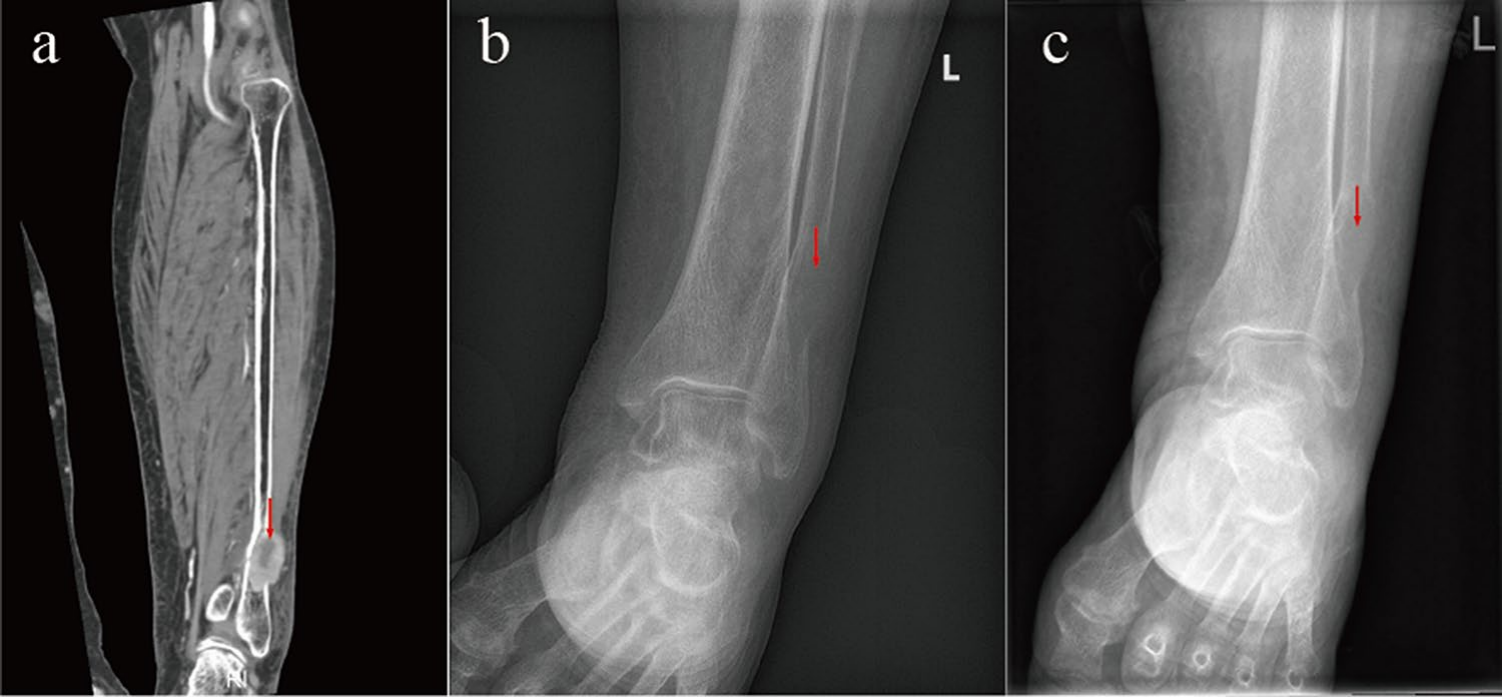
Imaging examination for Case 1. a MRI examination revealed a tumor in the distal left tibia. b X-ray examination showed a tumor in the distal left tibia. c X-ray examination showed that the left distal tibial tumor became smaller after operation
